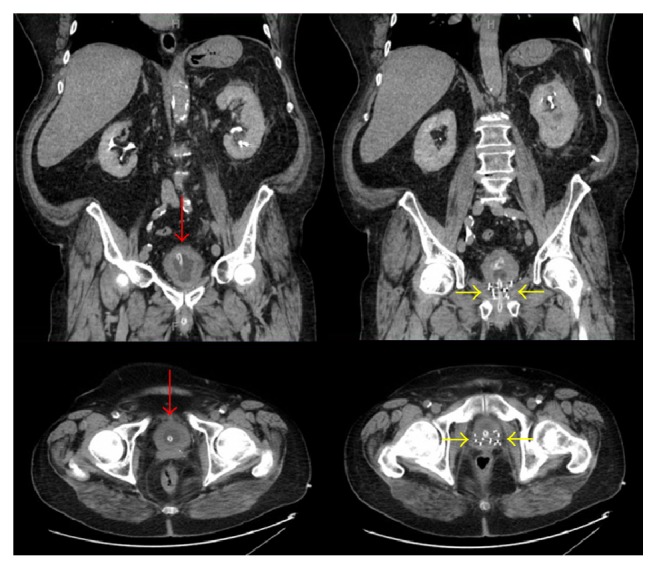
Computed tomography (CT) scan of the abdomen and pelvis revealed diffuse thickening of the wall of the urinary bladder with intravesical catheter* (red arrows)* and brachytherapy radiation seeds in the prostate* (yellow arrows)*.
